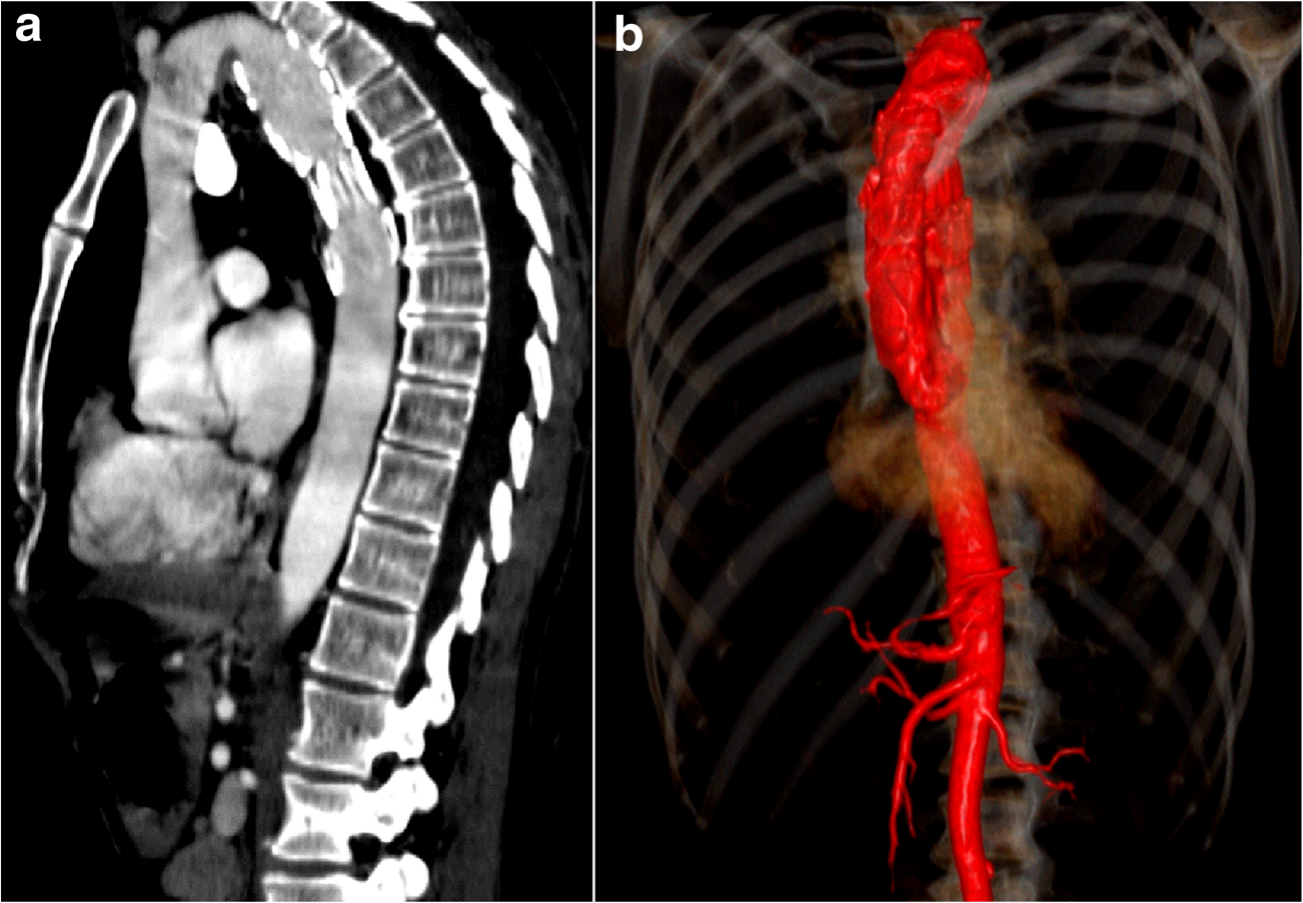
a, b A 69-year-old female with a remote history of treated small-cell carcinoma and stent graft placement of the descending aorta presenting with slowly progressive left supraclavicular mass. Axial contrast-enhanced CT (CECT) image (a) above the level of the sternoclavicular joint and volume-rendered image (b) identifies a high, dilated and elongated aortic arch extending into the left cervical region. These aortic arches can dilate with aneurysm formation, which can cause a mass effect on the surrounding structures in the thoracic inlet
